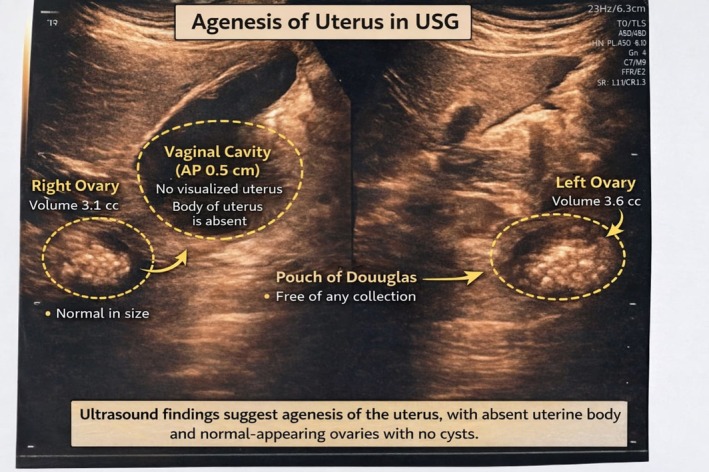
Pelvic ultrasonography of the patient. Transabdominal ultrasonography of the pelvis demonstrates key features of Müllerian agenesis in the present case. Uterus: Not visualized. Only a linear hypoechoic structure (anteroposterior diameter 0.5 cm) is seen, representing the blind vaginal pouch. Ovaries: Bilaterally normal in size and echotexture (Right ovary volume 3.1 cc, Left ovary volume 3.6 cc). Pouch of Douglas: Free of fluid collection. Additional findings: No appendicular or pelvic masses, ascites, pleural effusion, or lymphadenopathy detected. These findings demonstrate the absence of the uterine body with preserved ovarian morphology, correlating with clinical primary amenorrhea and supporting the diagnosis of complete Müllerian agenesis.
